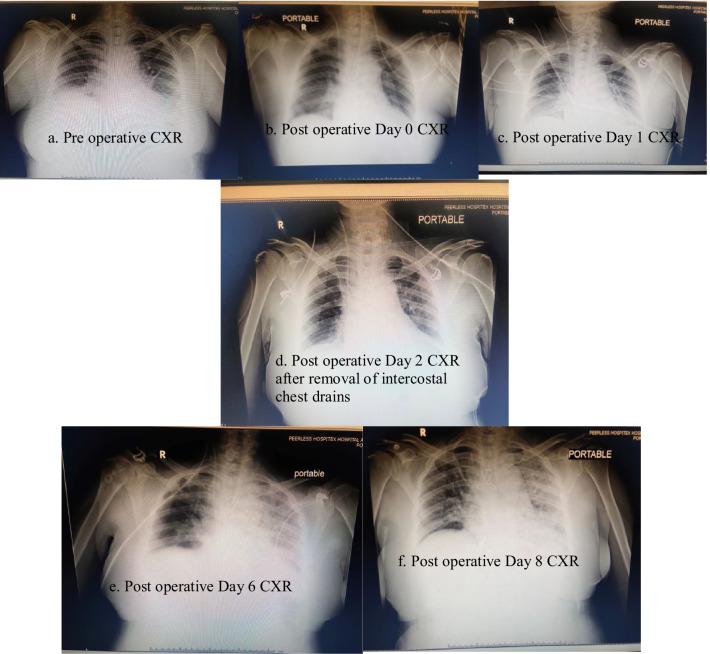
a–f A collage of chest X-rays (CXR) of the patient during her hospital stay
